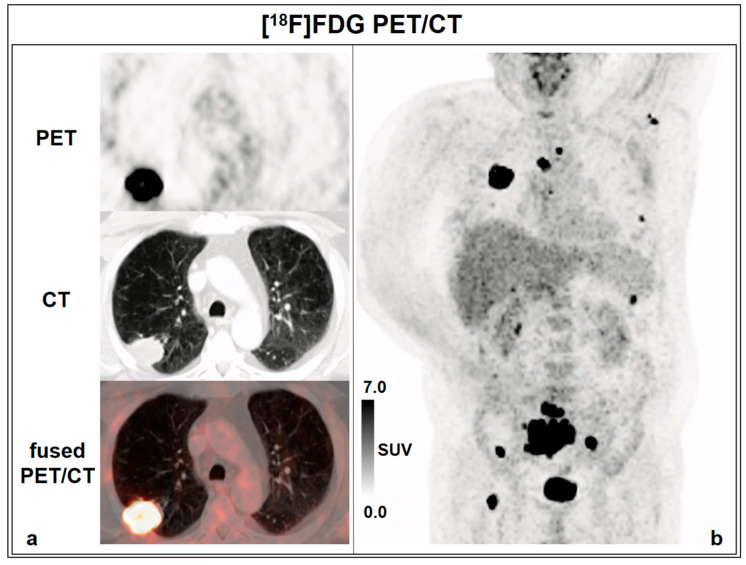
[^18^F]FDG PET/CT. (a) axial view. (b) maximum intensity projection (MIP).
